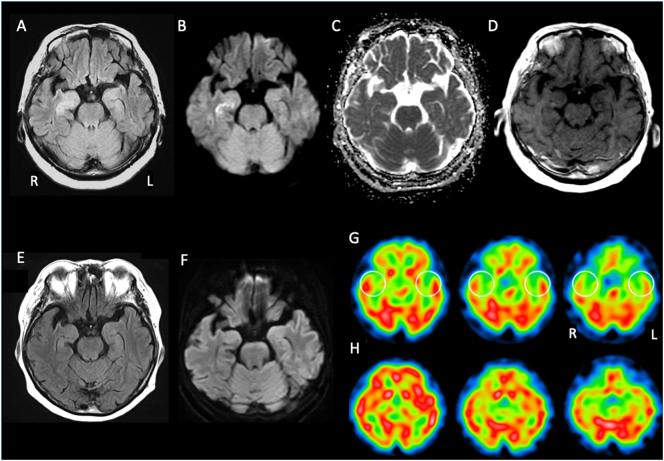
Brain MRI. (A) (B) The right dominant asymmetric hyperintensity involving the bilateral medial temporal regions can be seen in FLAIR and DWI images. (C) The ADC image shows no abnormal intensity corresponding to the hyperintense regions in DWI. (D) A post-contrast T1 image shows no gadolinium enhancement. (E) (F) The high intensity lesions in the medial temporal lobes have disappeared, as seen in FLAIR and DWI images after thymomectomy. Single photon emission computed tomography (SPECT) using I^123^-IMP. (G) Decreased blood perfusion in the bilateral medial temporal lobes can be seen (open circles). (H) Age-matched normal control.
